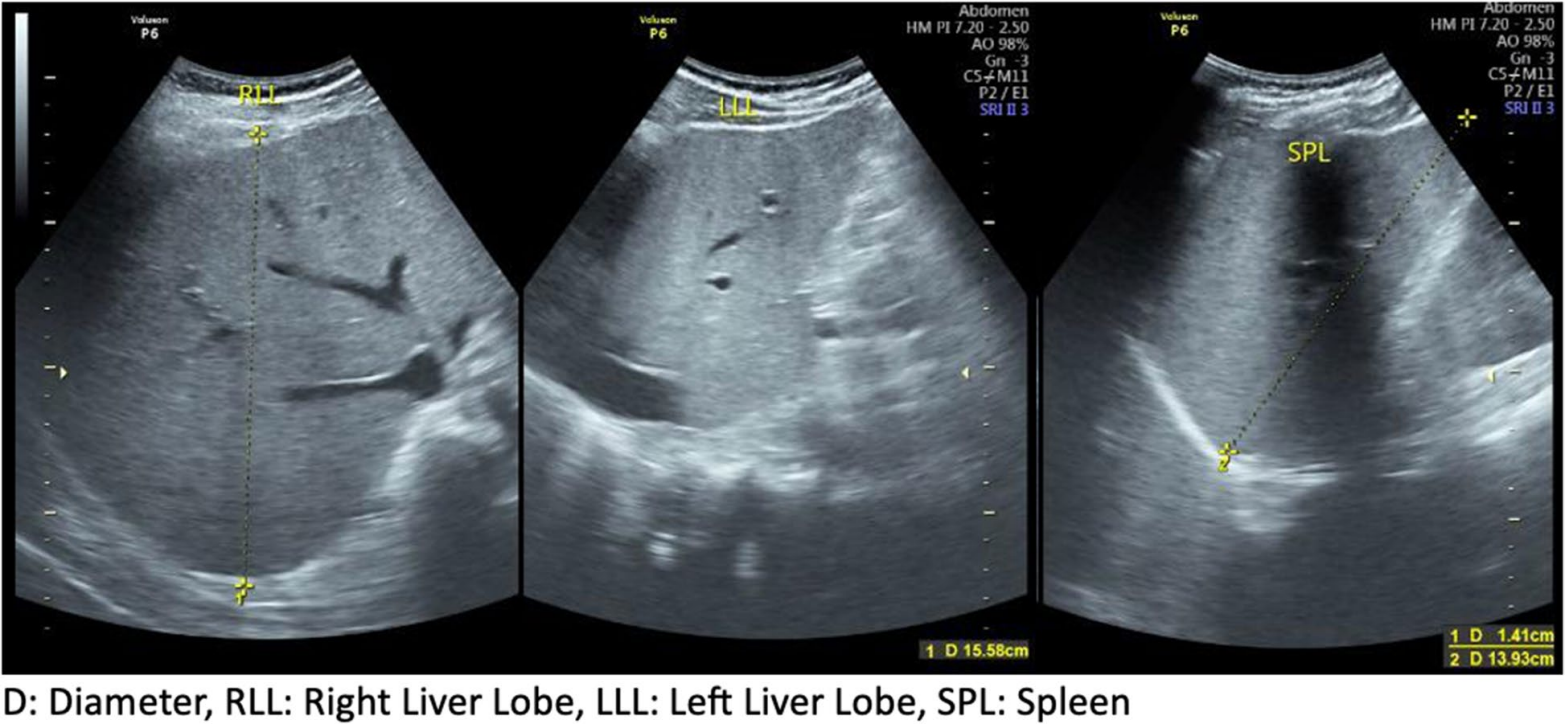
Abdominal sonography showing hepatosplenomegaly
